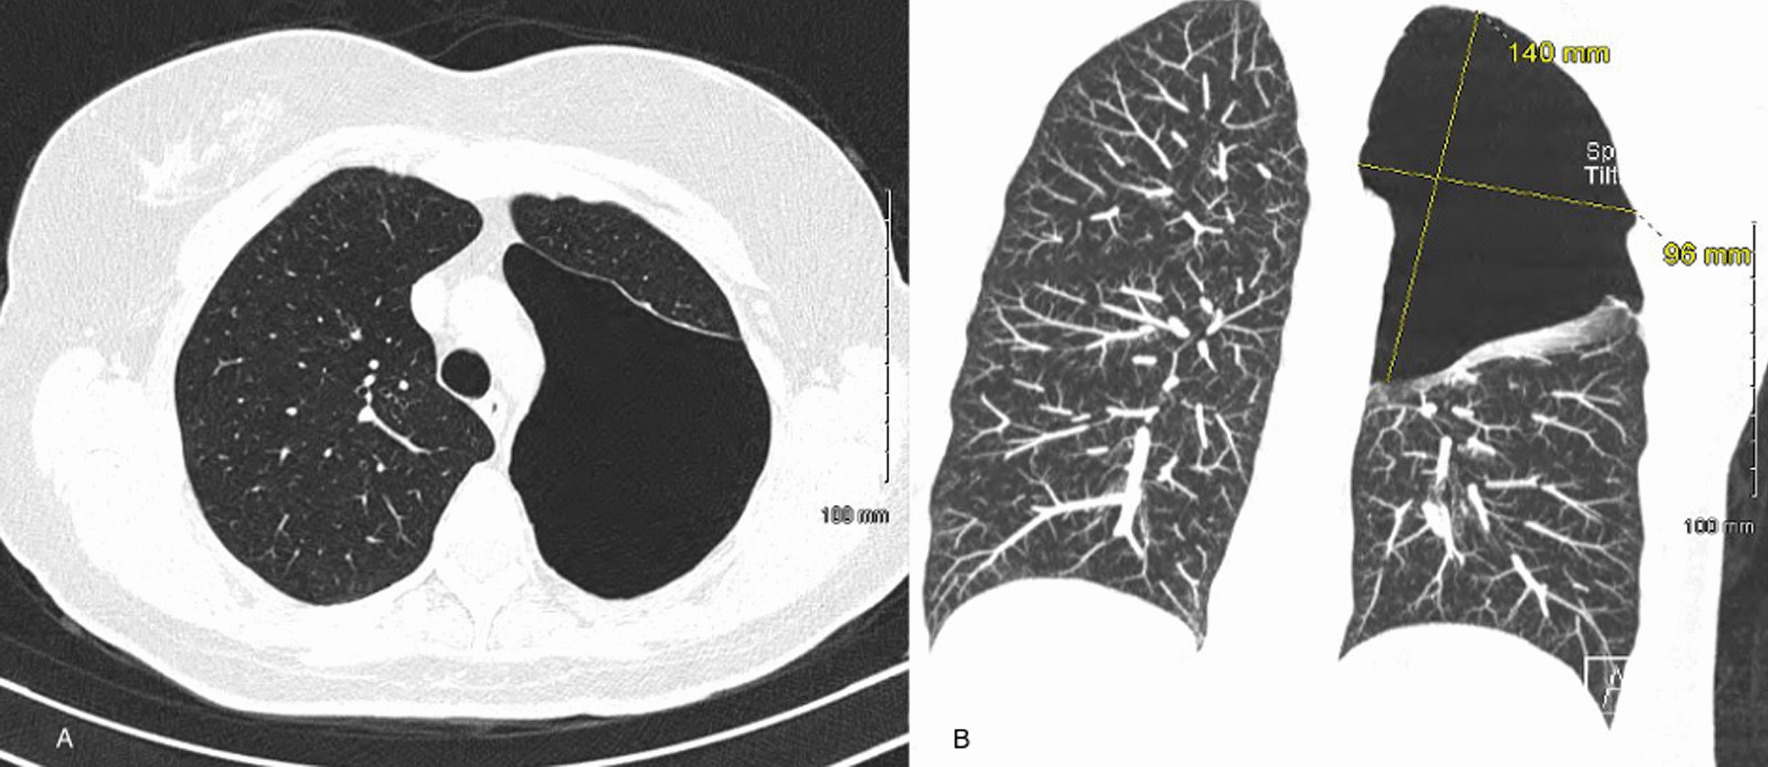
Initial CT imaging; a axial view, b coronal view. CT Imaging demonstrating a solitary giant bulla in the left upper lobe with normal surrounding parenchyma and secondary compression of the left upper and lower lobes
